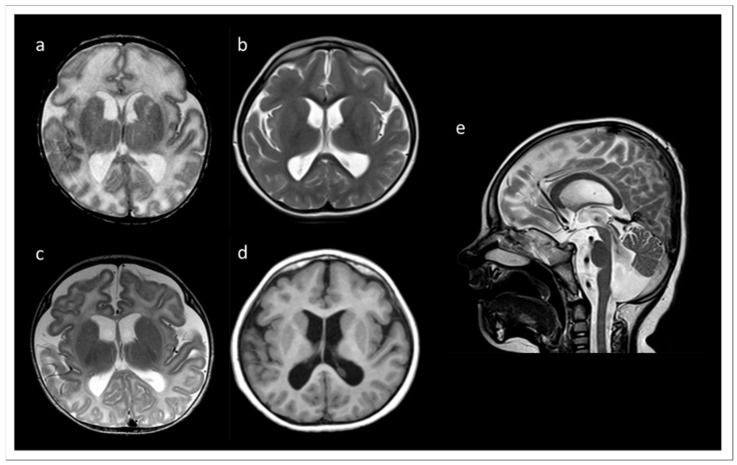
MRI records of the TARP-affected patient. (a) At term equivalent age the cerebrum was swollen with increased T2 signal of the supratentorial white matter and a simplified gyral pattern, especially frontal. In the further course, microcephaly with simplified gyral pattern and myelination delay were visible. (b) T2 at the age of 3 months. (c) T2 at the age of 3.5 years. (d) T1 at the age of 3.5 years. (e) T2 at 3.5 years of age showing concomitant ventriculomegaly, thickening of the corpus callosum, and pontine and vermis hypoplasia.
